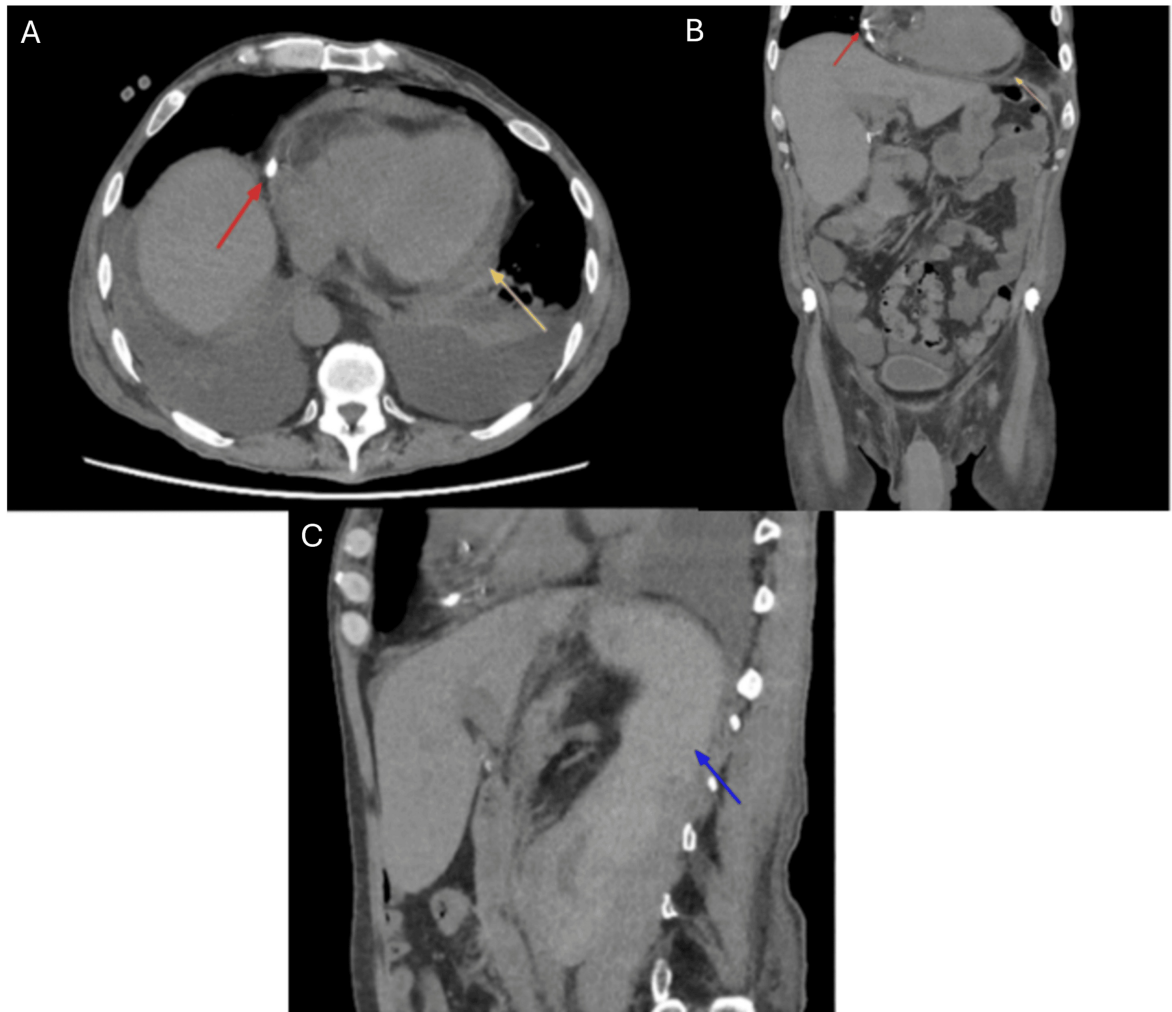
CT scan revealing intrapericardial malposition of the catheter. (A-C) CT scan showing intrapericardial malposition of the catheter (red arrow) associated with minimal pericardial effusion (yellow arrow). The retroperitoneal hematoma (blue arrow) appears stable. (A) Axial view; (B) Coronal view; (C) Sagittal view.
